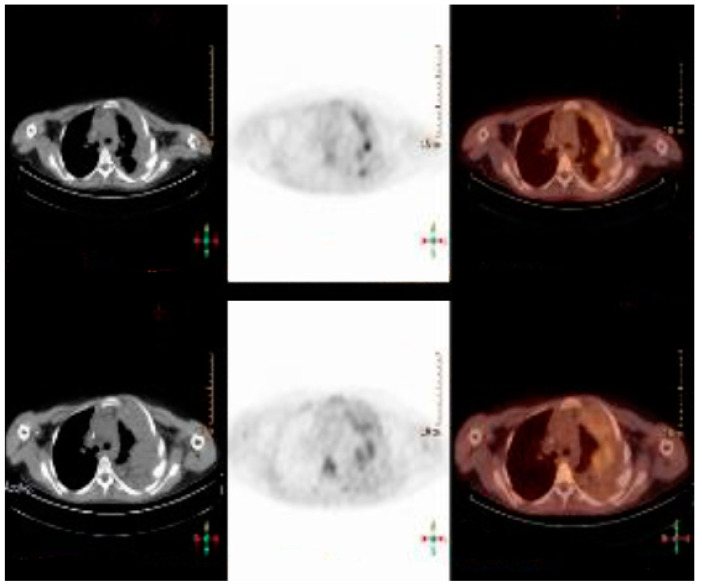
FDG-PET obtained after the fourth cycle of CT.
